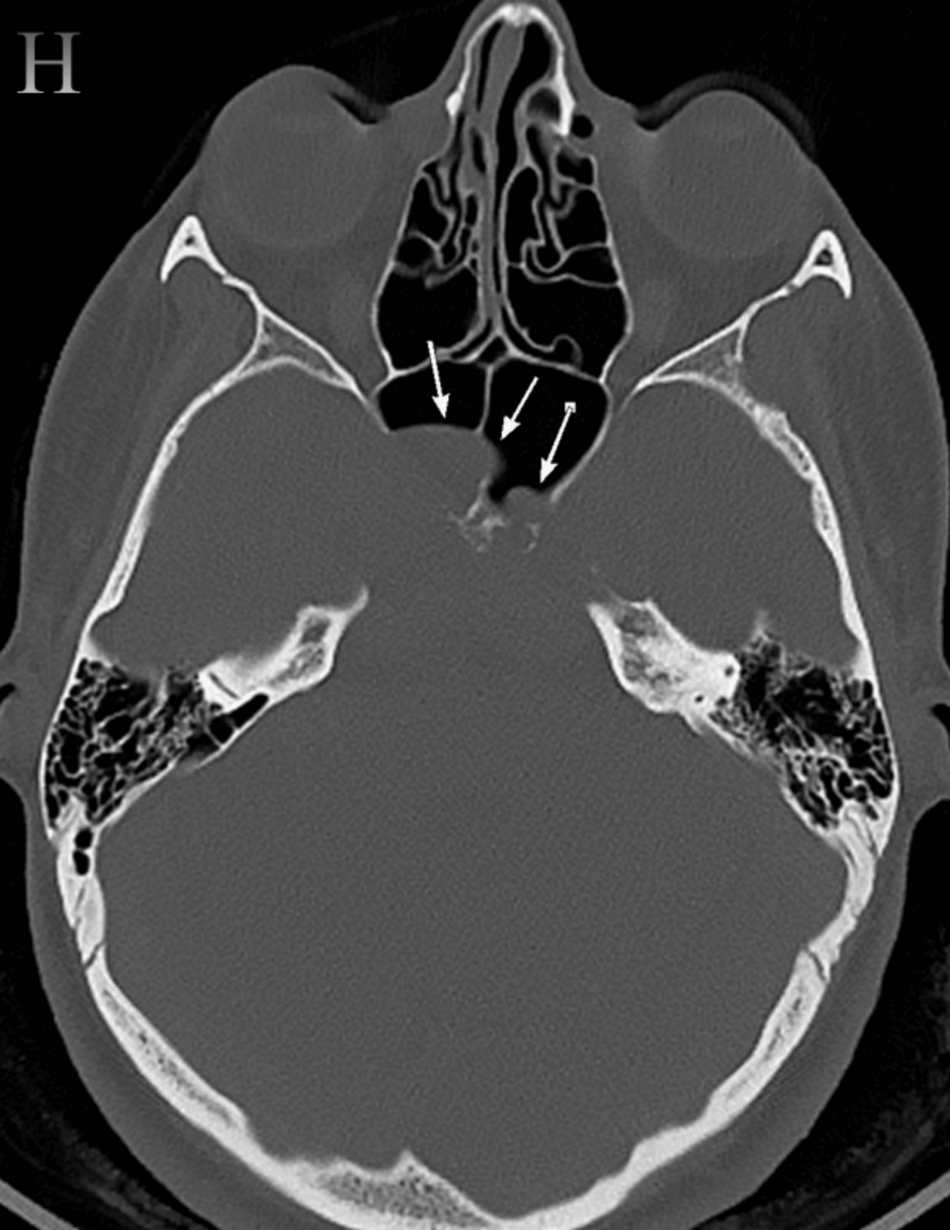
Bone window axial CT (H) shows extension into the sphenoid sinus (long arrows) CT: computed tomography
